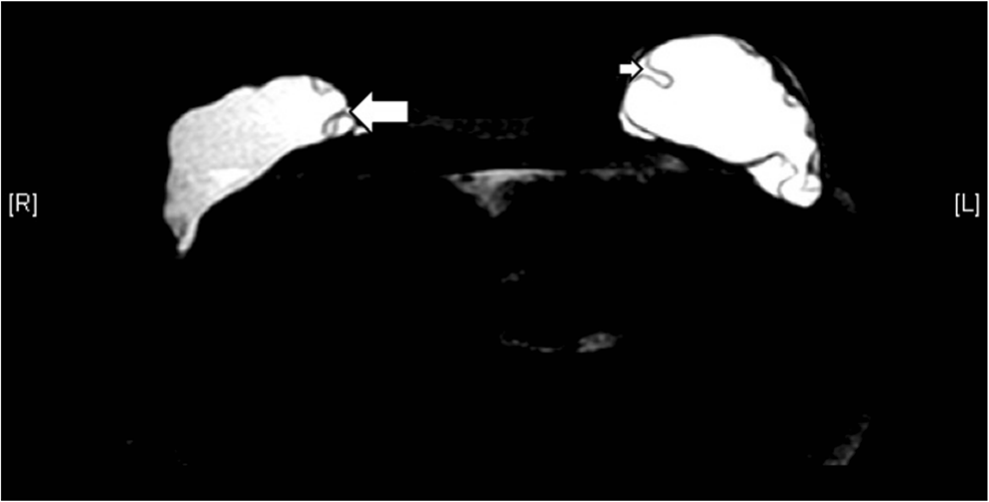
Keyhole sign (left breast) and teardrop sign (right breast). Silicone-only axial MR image shows keyhole sign (small arrow) at left breast. The invaginations of implant shell do not touch each other. Tear-drop sign is seen at right breast (large arrow), with the invaginated membranes contacting one another“Tear-drop sign” differs from “keyhole sign” in that the invaginated membranes contact one another (Fig. 10).“Linguine sign” is the presence of curvilinear hypointense lines, which represents the collapsed shell, floating in the high-signal intensity silicone gel [3] (Fig. 11). This sign is only present in collapsed intracapsular rupture.
